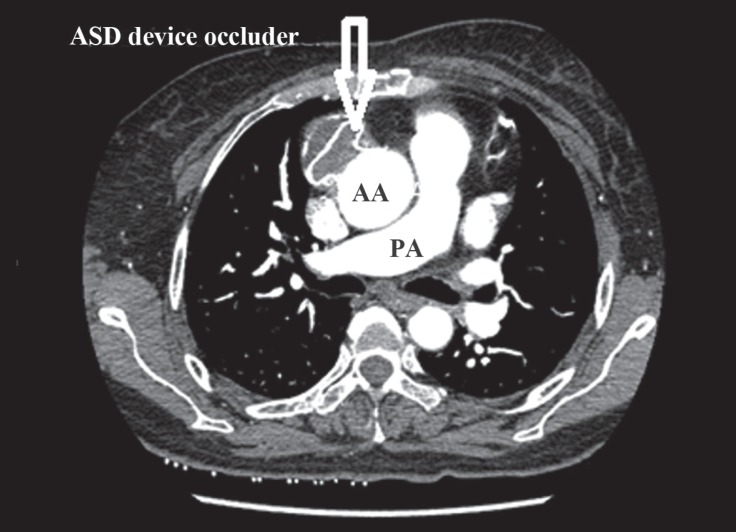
Postprocedural multidetector computed tomographic angiography shows the atrial septal defect (ASD) device occluder in the appropriate site and no leakage.
